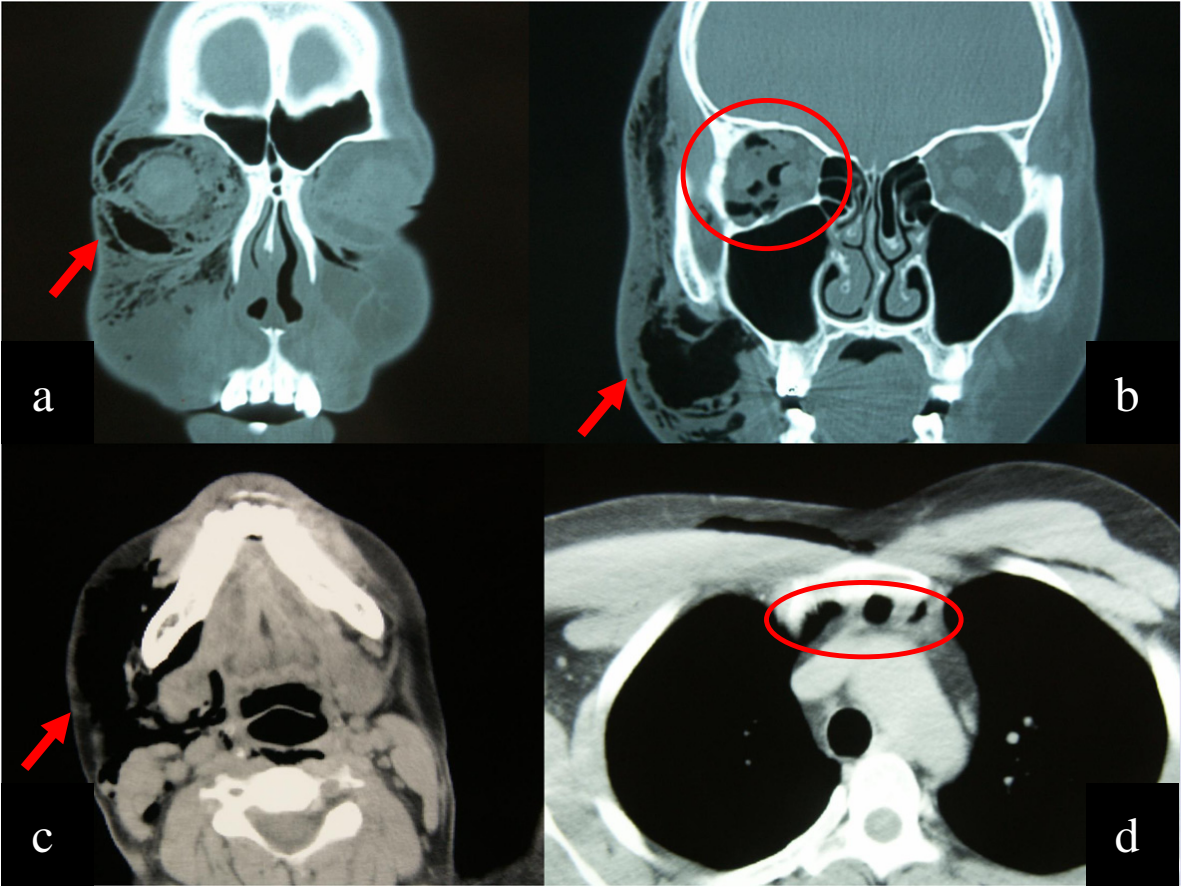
An emergency computed tomography (CT) scan of the orbit, brain, neck, and chest. (a) The CT demonstrated subcutaneous emphysema of the right eyelids (arrow). (b) Emphysema in the right orbit was observed (circle). Subcutaneous emphysema of cheek area was also found (arrow). There was no apparent air in the intracranial space and fracture of the orbital wall or floor. (c) The existence of subcutaneous air in the neck was also confirmed (arrow). (d) Mediastinal emphysema was also found (arrow).
